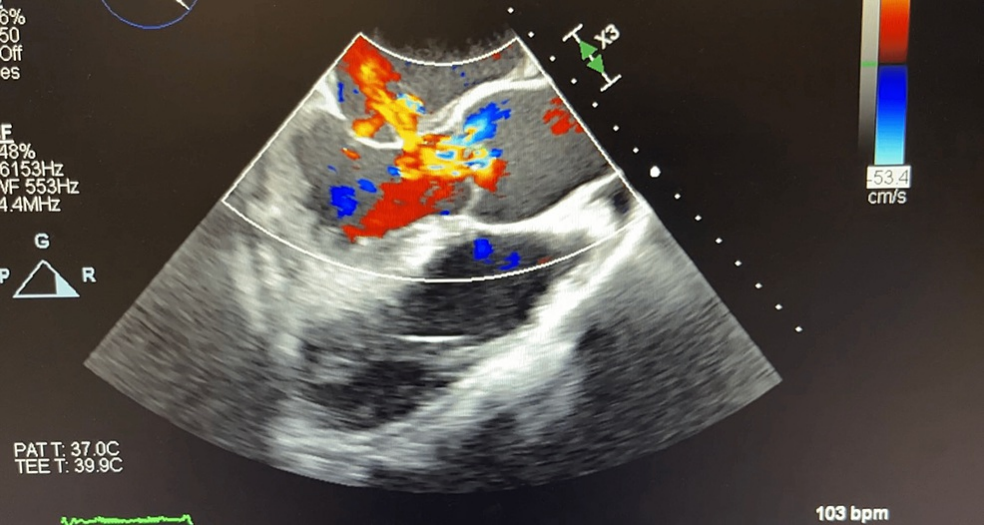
Transesophageal Echocardiogram Showing Severe Aortic Regurgitation, Diastolic Mitral Regurgitation, and Anterior Mitral Leaflet Perforation
